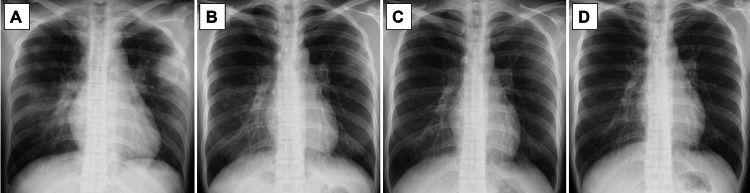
Chest radiography Chest radiography demonstrating progressive improvement of pulmonary infiltrates over time. (A) Chest radiography on day 1. (B) Chest radiography on day 5. (C) Chest radiography on day 10. (D) Chest radiography on day 12.
